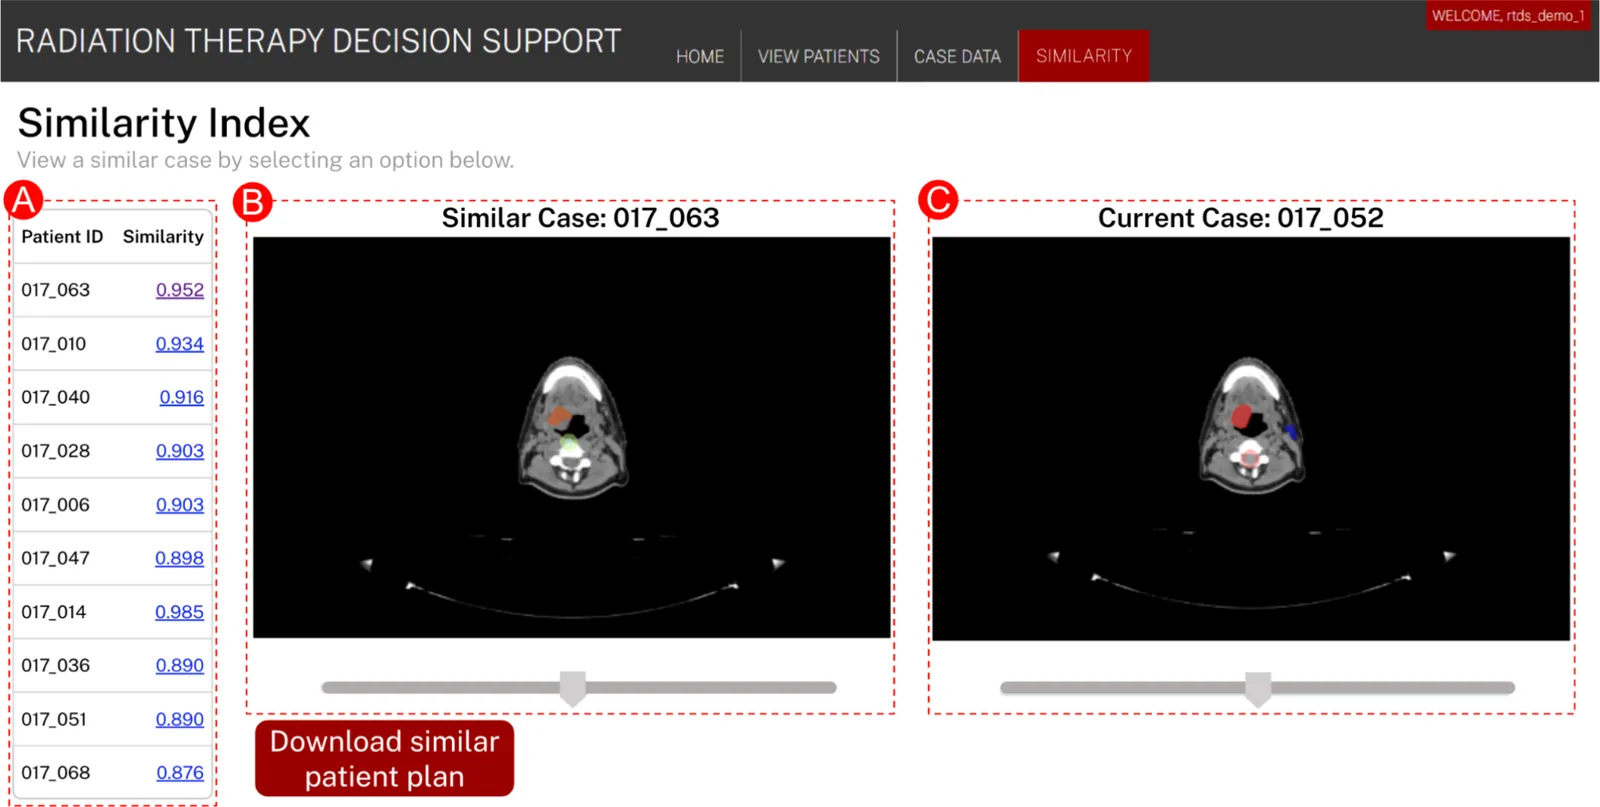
Similarity Index Viewing Page. Figure showing web application similarity viewing page. a Similarity index showing the ten most similar retrospective cases and their similarity. b CT Image slider of the currently selected similar retrospective case. c CT Image slider of the current planning case
